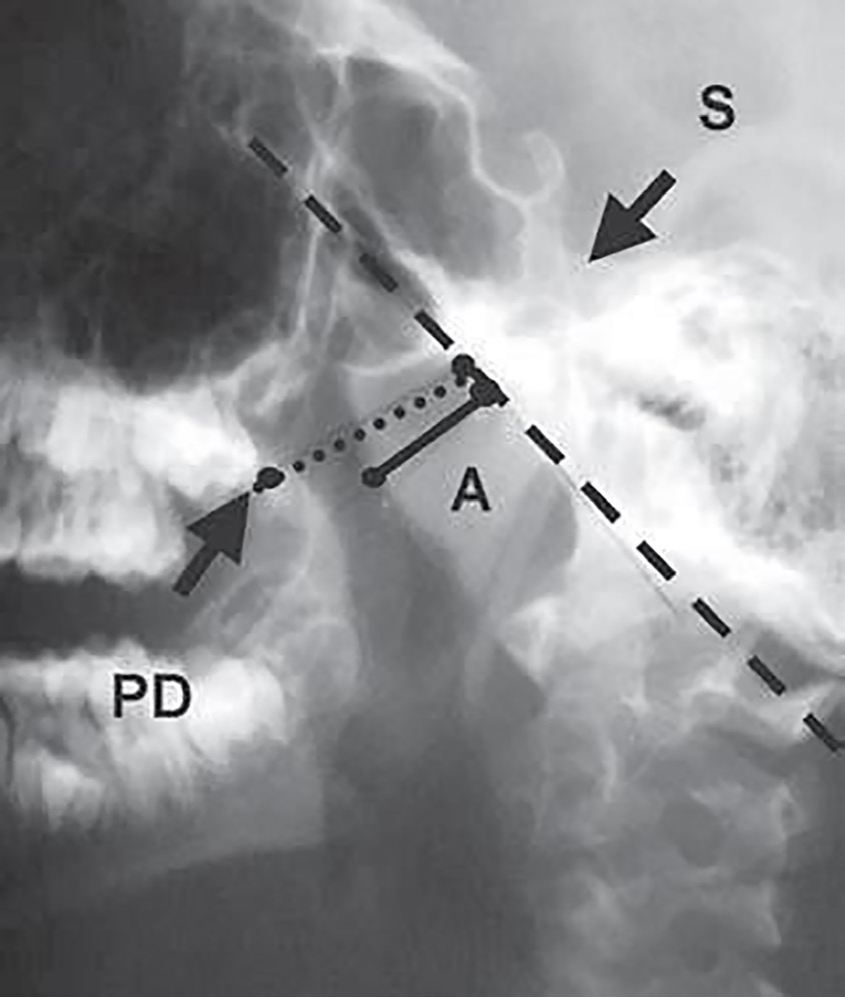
Cavum X-Ray - adenoid nasopharynx ratio (ANR) – black line is the measure of the adenoid / dotted line is nasopharynx measure; ANR is the result of the division of the measures of the black line by the dotted line.
